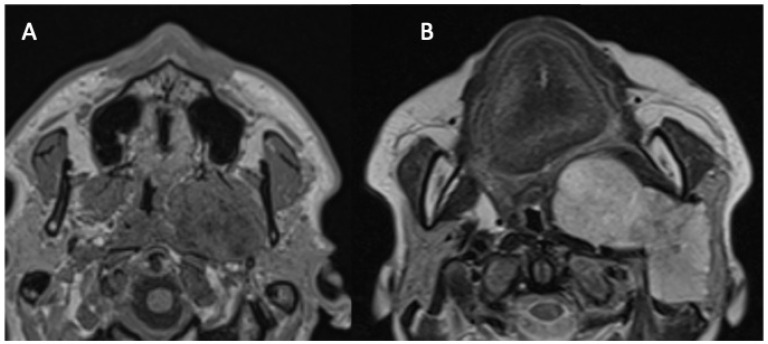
Two forms of the parapharyngeal space involvement of parotid tumors. Magnetic resonance images: axial T1WI images at the level of the hard palate. (A) Non-contrast image presenting an isolated PPS tumor developed from the medial protuberance of the parotid gland deep lobe. (B) Contrast-enhanced image showing a deep lobe tumor with the extensive involvement of the PPS.
